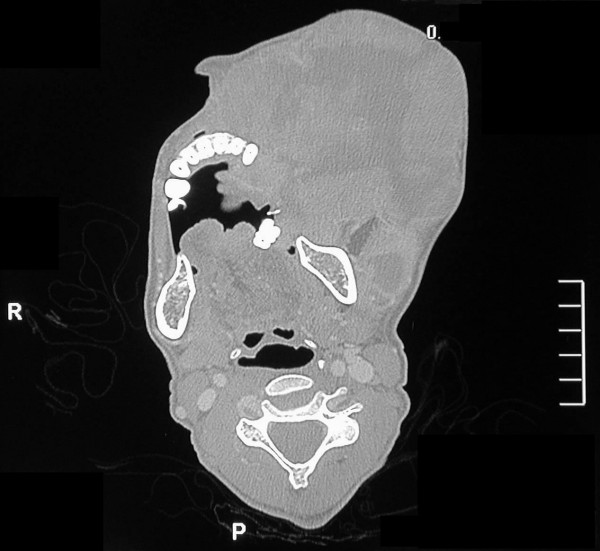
Axial CT cut showing the invasion of the floor of the mouth by the tumour.
